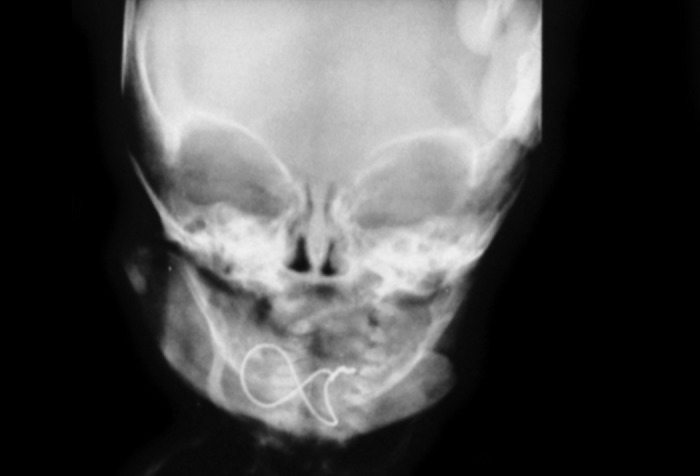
Postoperative radiograph with figure of eight wiring
